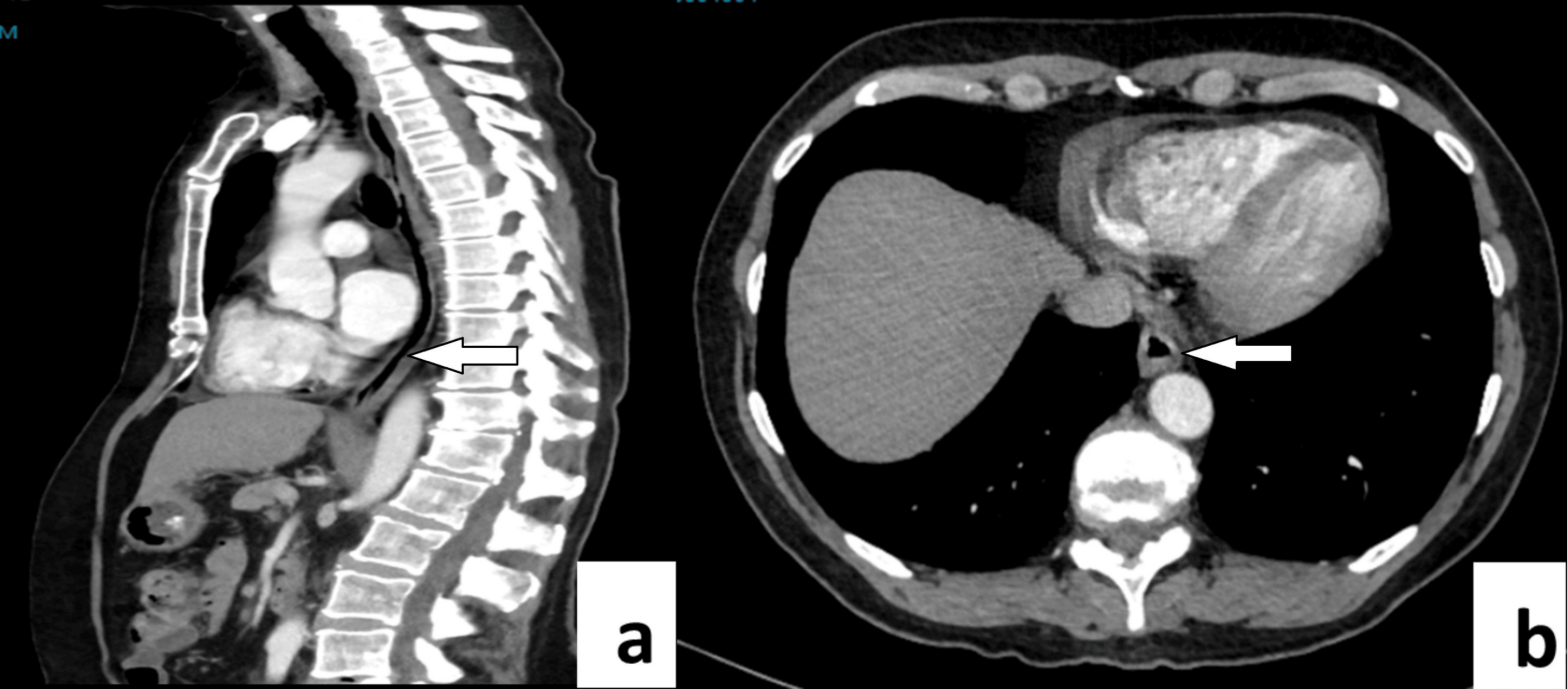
Post-operative Computed Tomography (CT) scan Post-operative Computed Tomography (CT) scan (a. sagittal, b. axial views) 12 months post robotic-assisted minimally invasive resection of multiple oesophageal schwannomas. White arrows indicate the site of the previous distal oesophageal mass.
